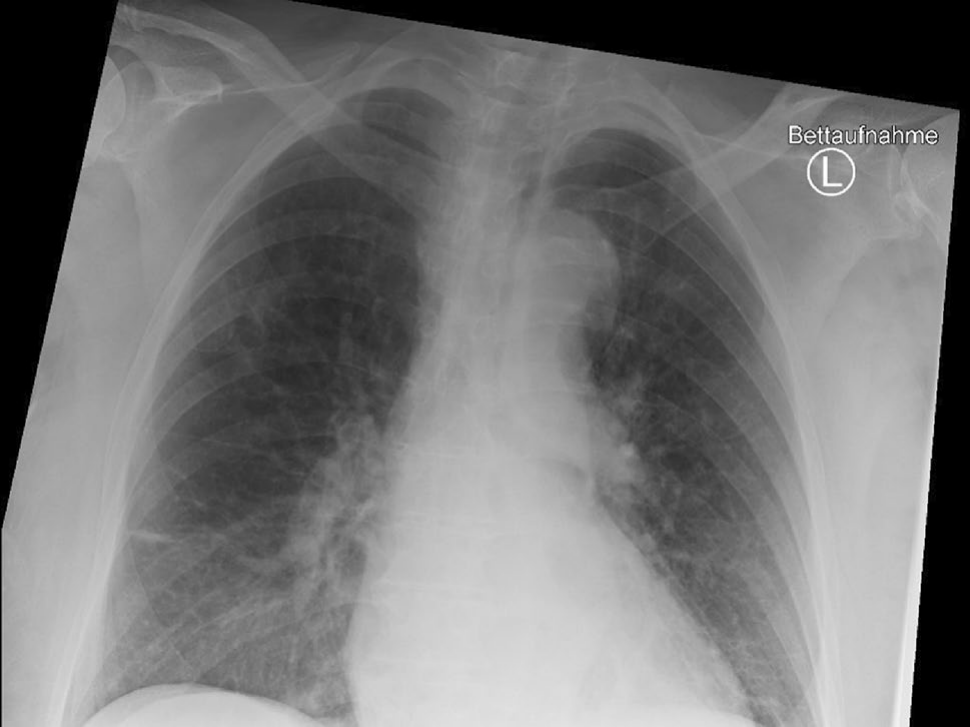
Chest X-ray on admission. Chest X-ray on admission showed basally accentuated bipulmonary infiltrates with signs of incipient pulmonary venous congestion
